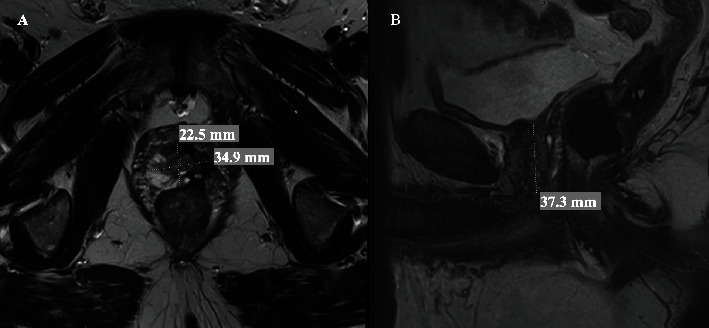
Axial (A) and sagittal (B) views of the multiparametric prostate MRI showing the prostate gland measurements with significant atrophy and total volume of 17 g.
